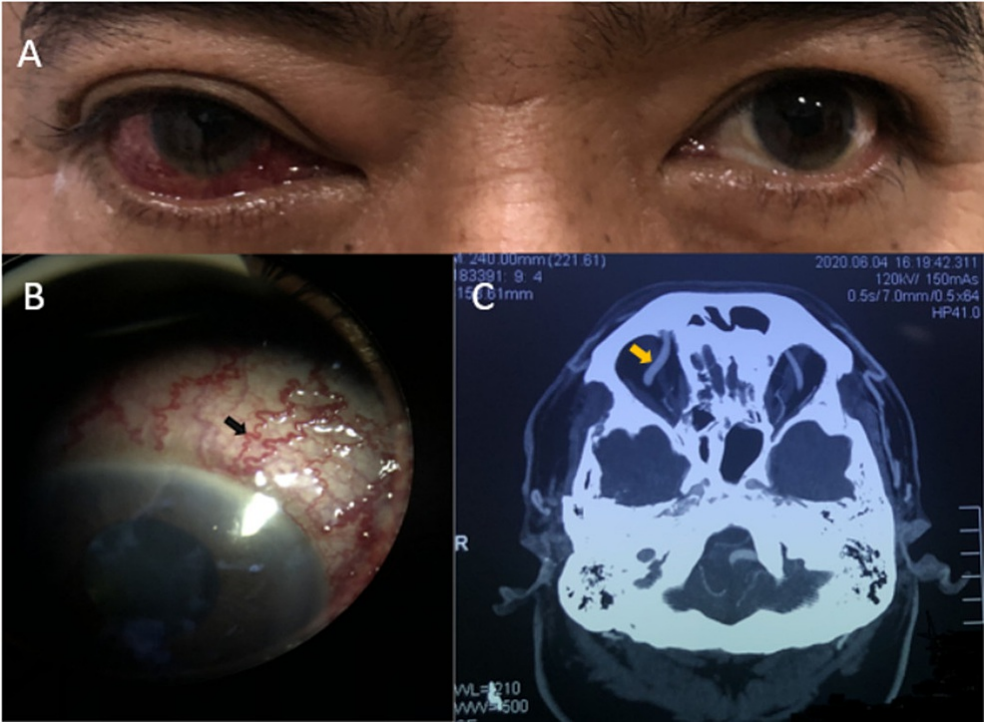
Ocular and radiological findings in Case 1. (A) Right eye redness with lid swelling. (B) Corkscrew vessels (black arrow) on slit-lamp examination. (C) Dilated right SOV (yellow arrow) in CTA.
